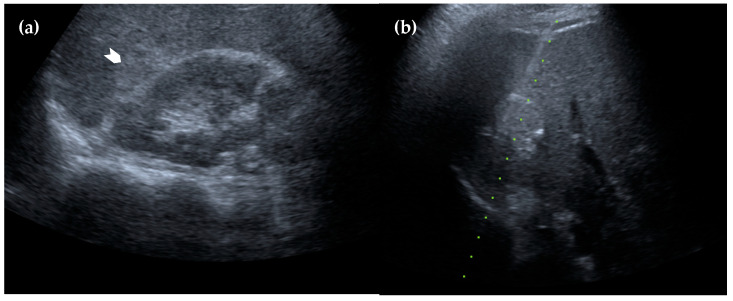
Ultrasonography of the liver. (a) The lesion appears homogeneously hyperechoic with a well-defined margin (white arrowhead); (b) an ultrasound-guided biopsy was performed, and the pathology results confirmed the diagnosis of intrahepatic splenosis.
